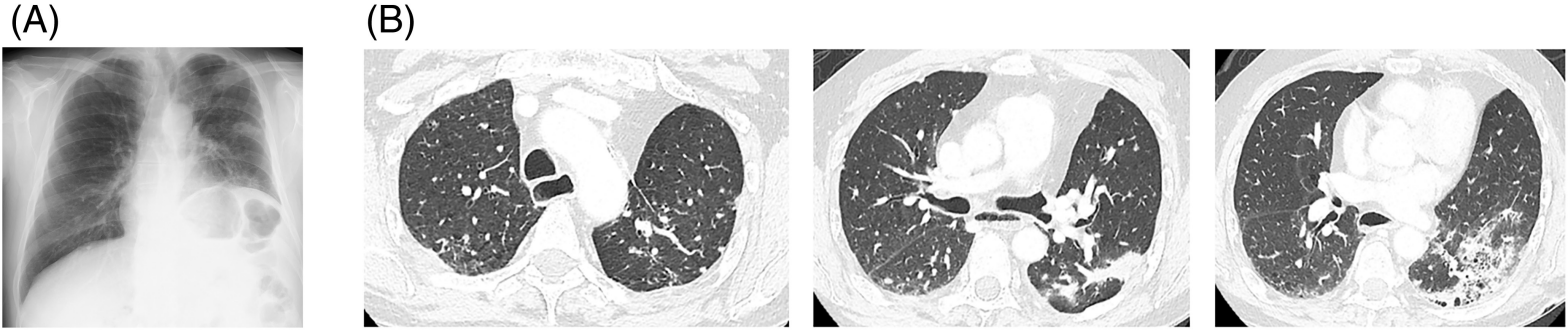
Chest x‐ray and computed tomography before anamorelin. (A) Chest x‐ray showed volume reduction in the left lung due to a previous left upper lobectomy. (B) Chest computed tomography revealed consolidation in the left lower lobe along with bilateral multiple pulmonary metastases.
